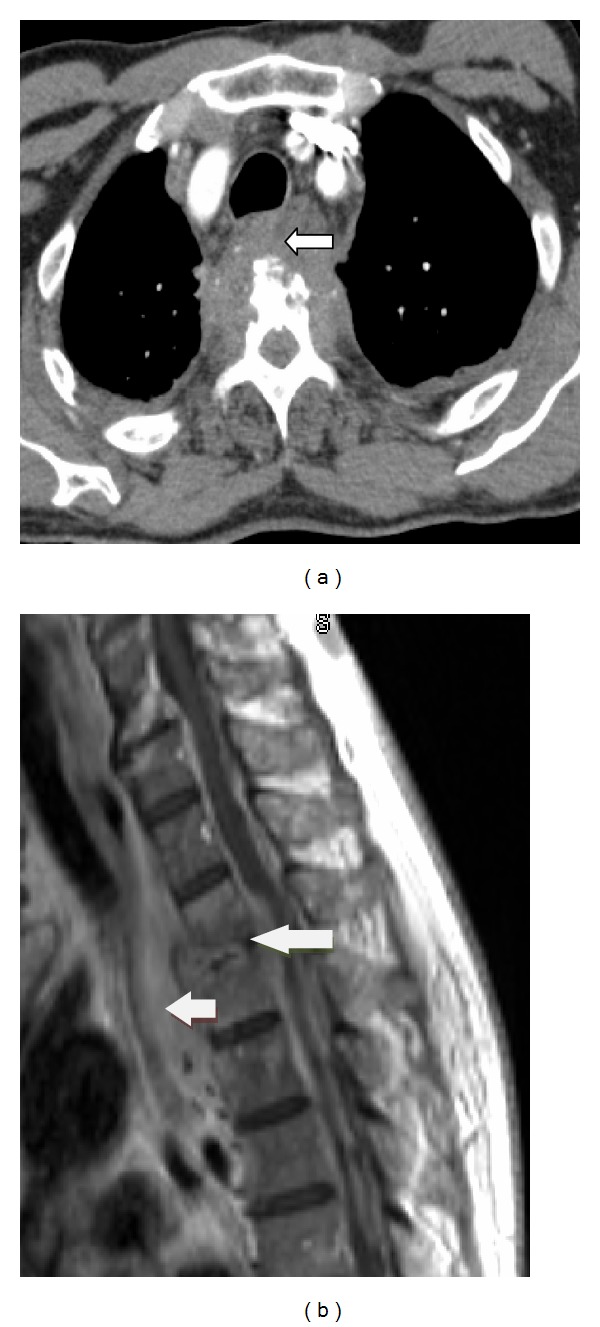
(a) CT angiogram chest showing a large soft tissue mass at the T3-T4 vertebral space with associated osseous destruction of the T4 vertebral body. (b) MRI of the thoracic spine, T1 weighted, showing T3-T4 disk space narrowing, endplate destruction, and marrow edema. There is also a soft tissue mass extending from the space anteriorly in the prevertebral space.
